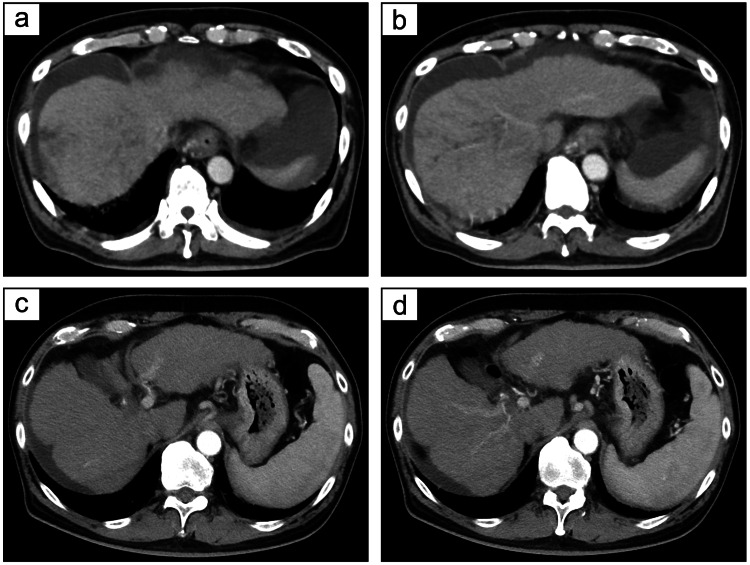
Contrast-enhanced computed tomography scan before and after treatment (a, b) Contrast-enhanced computed tomography (CT) showing a 37-mm mass in segment 8 of the liver with a tumor thrombus in the right portal vein branch. The lack of early contrast enhancement in the dynamic study suggests poorly differentiated hepatocellular carcinoma. (c, d) Follow-up contrast-enhanced CT images showing disappearance of the segment 8 liver mass, indicating a complete response.
